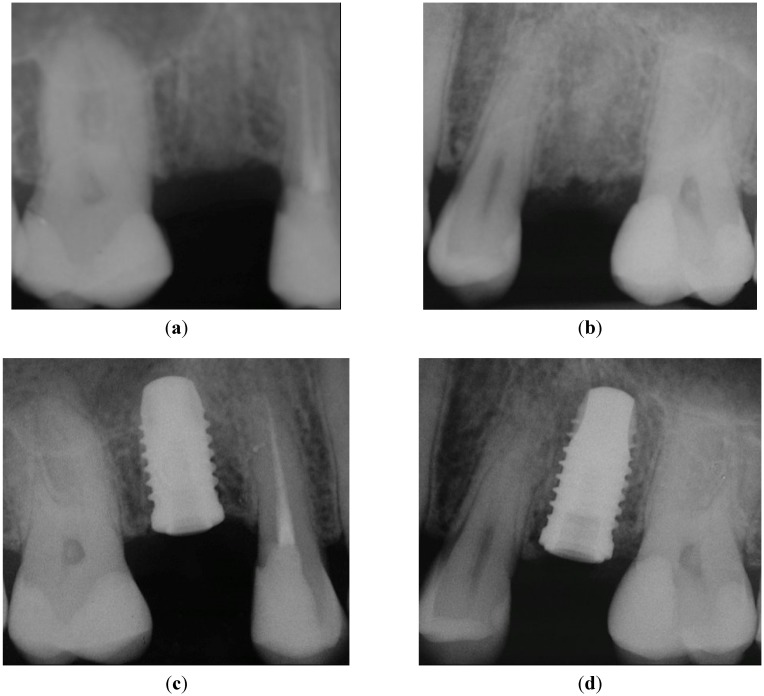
X-rays taken before implant insertion and immediately after implant placement: (a) healed crestal bone in Position 15; (b) healed crestal bone in Position 25. No pathological signs were present after implant placement of both implants: (c) Position 15, implant with PFS; (d) Position 25, StE implant.
